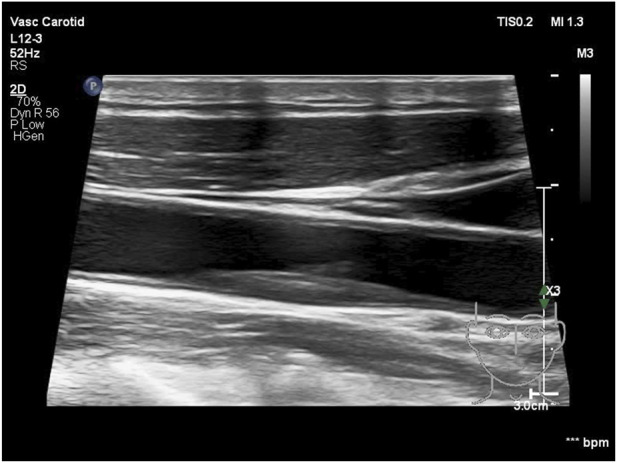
Axial dynamic ultrasound image of the carotid artery bifurcation.
